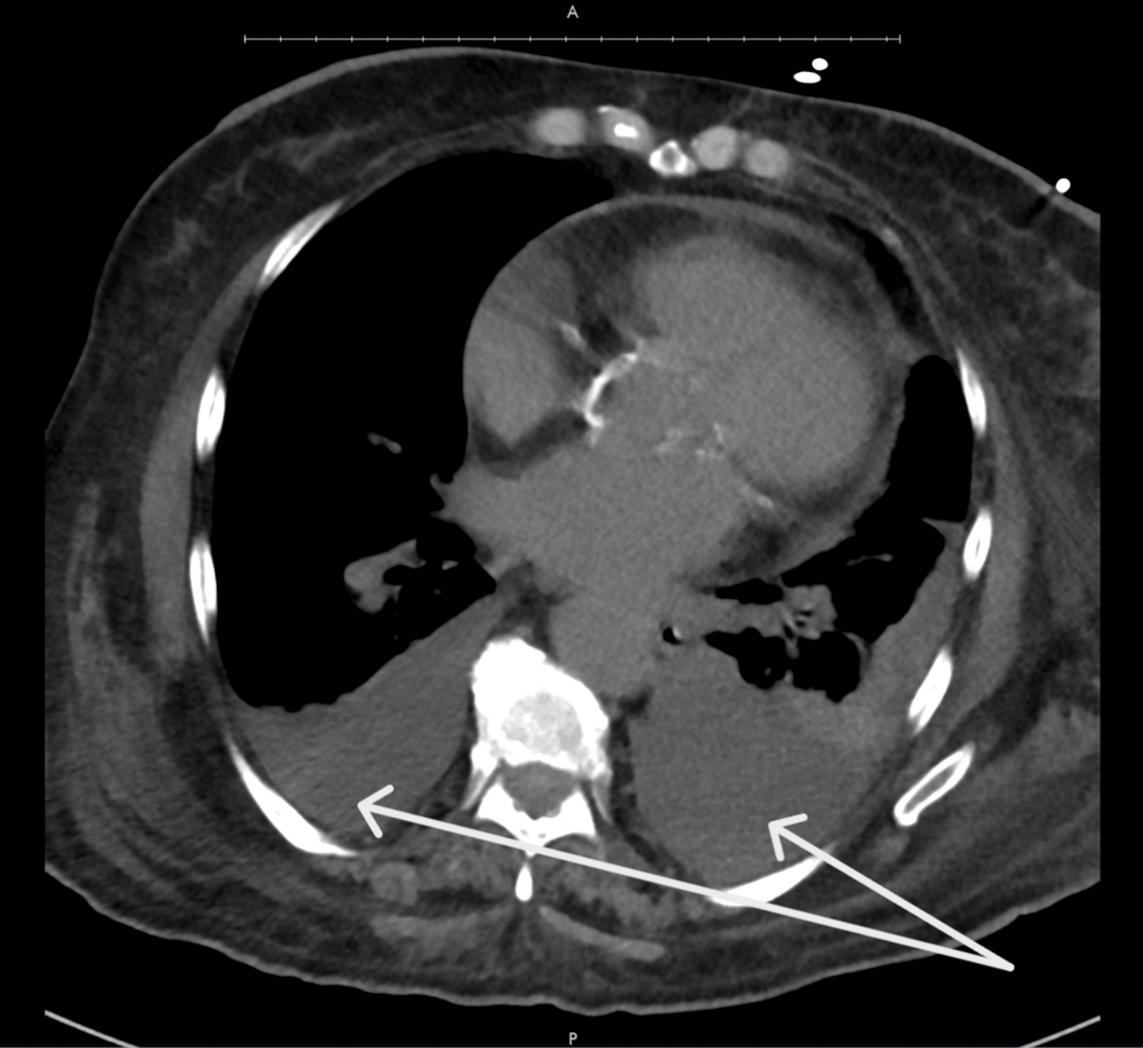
CT Chest. Note the Moderate Bilateral Effusions With Atelectasis and Small Pericardial Effusion.
